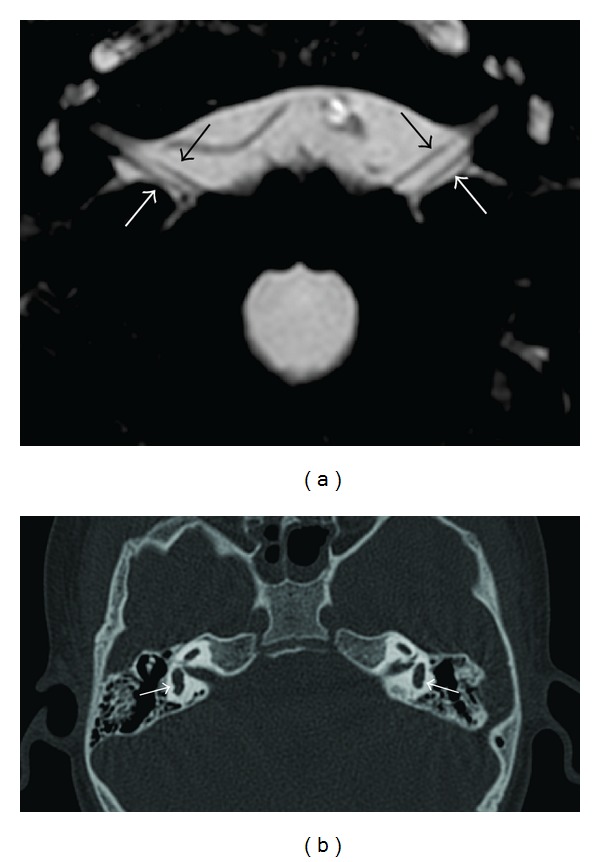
(a) Axial cross-sectional MRI 3D CISS sequence with mean intensity projection through the IAC and cerebellopontine angle. Only two nerves are detected: the facial nerve (black arrow) and the vestibular nerve (white arrow). (b) Axial CT image through the mastoid shows vestibules with dysplastic morphology (white arrow). Note the absence of semicircular canals.
